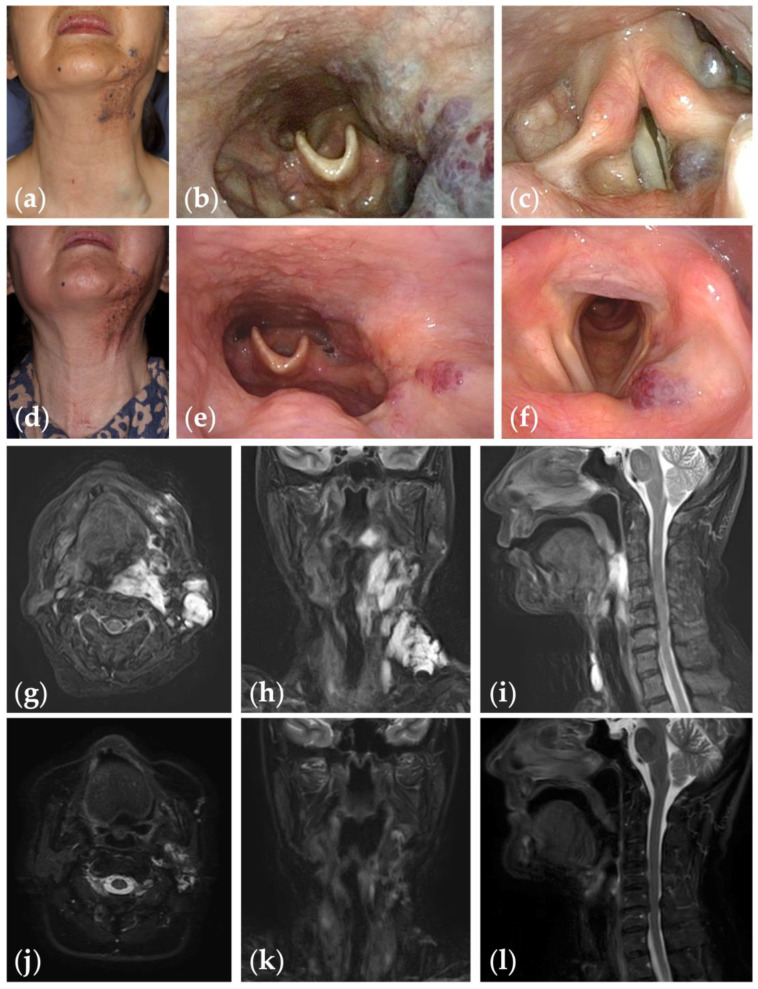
Patient 1 graded “excellent” for treatment outcomes after nine sessions of fluoroscopy- and endoscopy-guided transoral sclerotherapy: (a) initial clinical photograph, (b) endoscopic photographs of the pharynx; and (c) the glottis of a 67-year-old woman with oropharyngolaryngeal venous malformation; (d) clinical photograph; (e) endoscopic photographs of the pharynx; and (f) the glottis six months after the final sclerotherapy session at the age of 75 years; (g–i) fat-suppressed T2-weighted magnetic resonance images before treatment; (j–l) short tau inversion recovery T2-weighted magnetic resonance images six months after the final sclerotherapy session.
